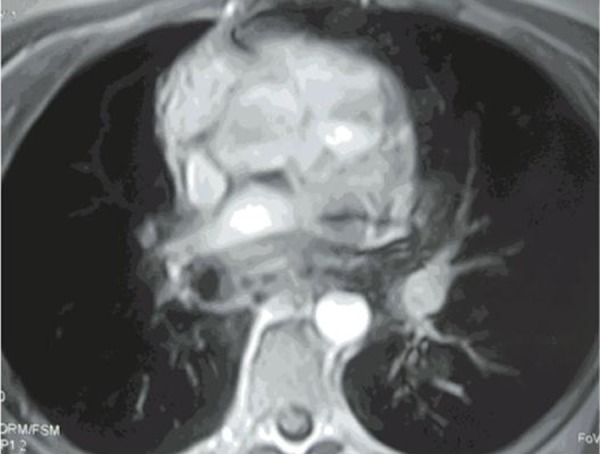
Post-treatment, follow-up, axial T1-weighted, post gadolinium image. The
